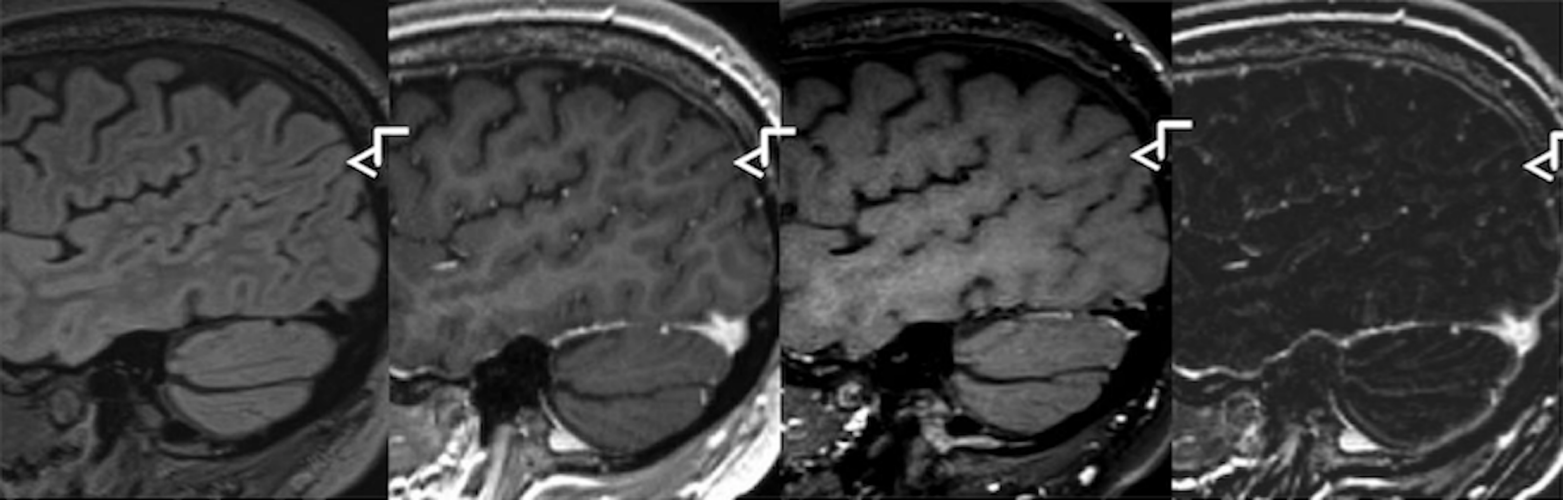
Improved visibility of a juxtacortical lesion in T1w dark blood image. From left to right: FLAIR, T1w-MPRAGE after Gadolinium injection, T1w dark blood after Gadolinium injection, subtraction image.
